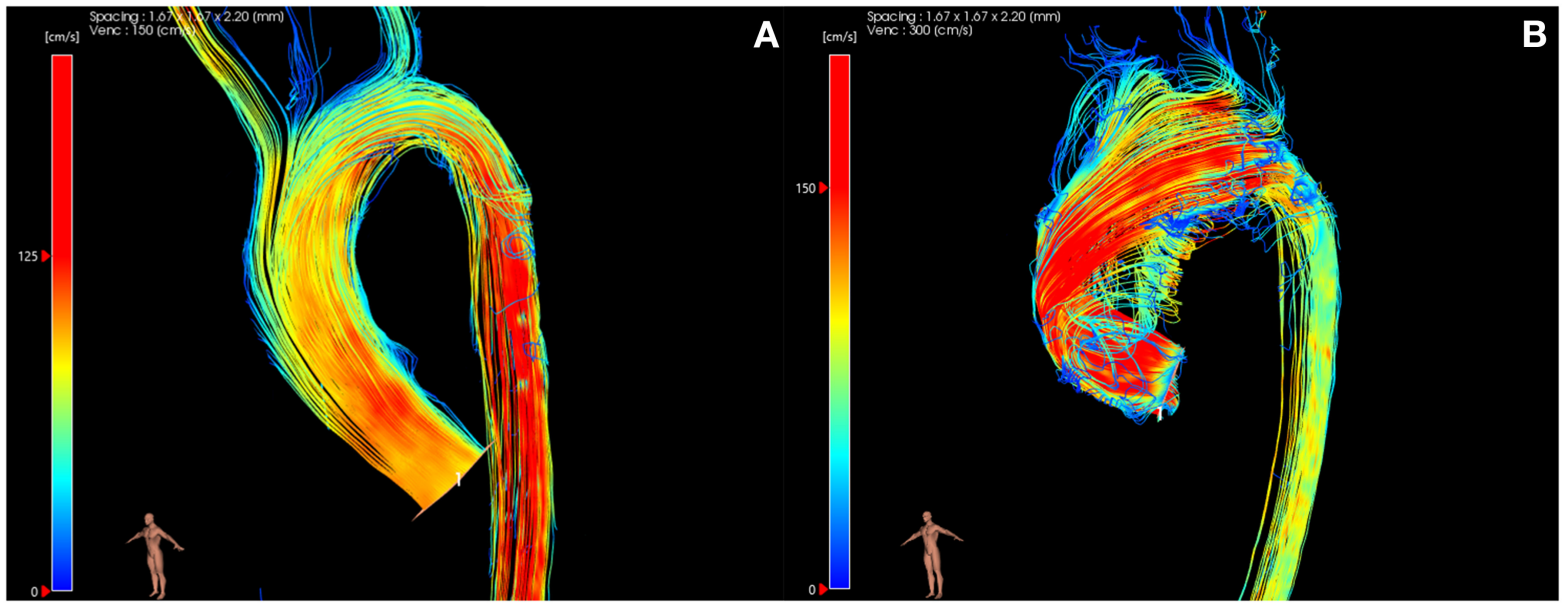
4D flow MRI images showing right-handed helical flow in normal (A) and BAV (B) subjects.
